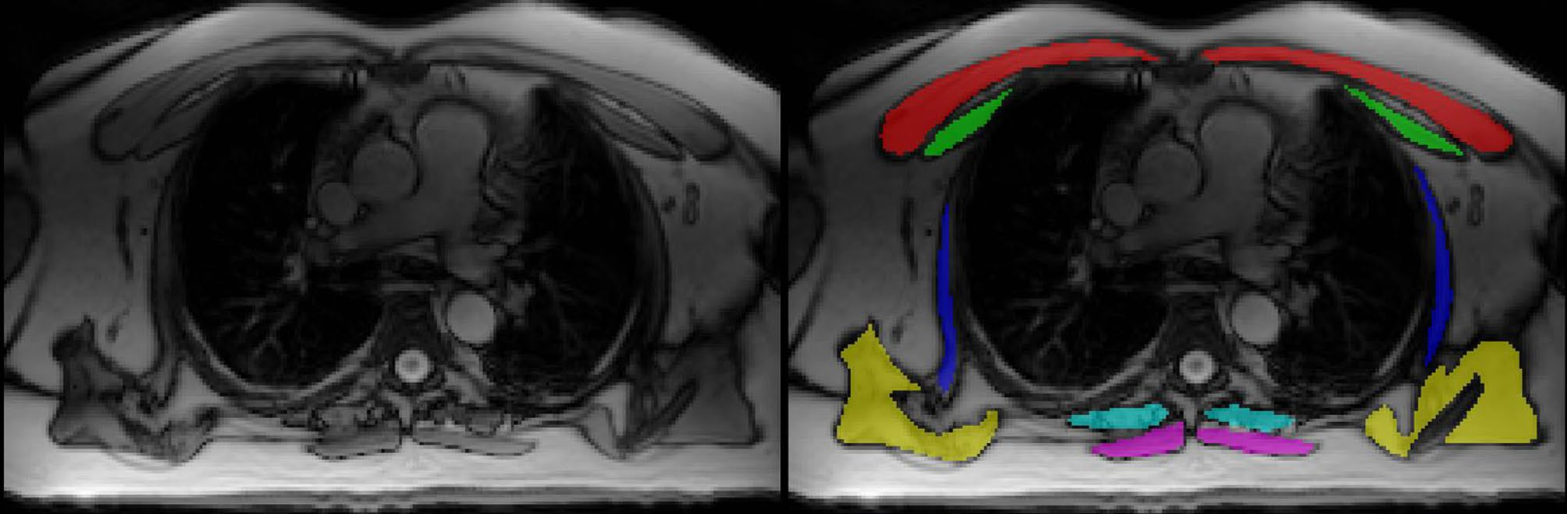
Axial MRI image at the level of carina demonstrating the measurements of bilateral cross-sectional area of thoracic skeletal muscle
